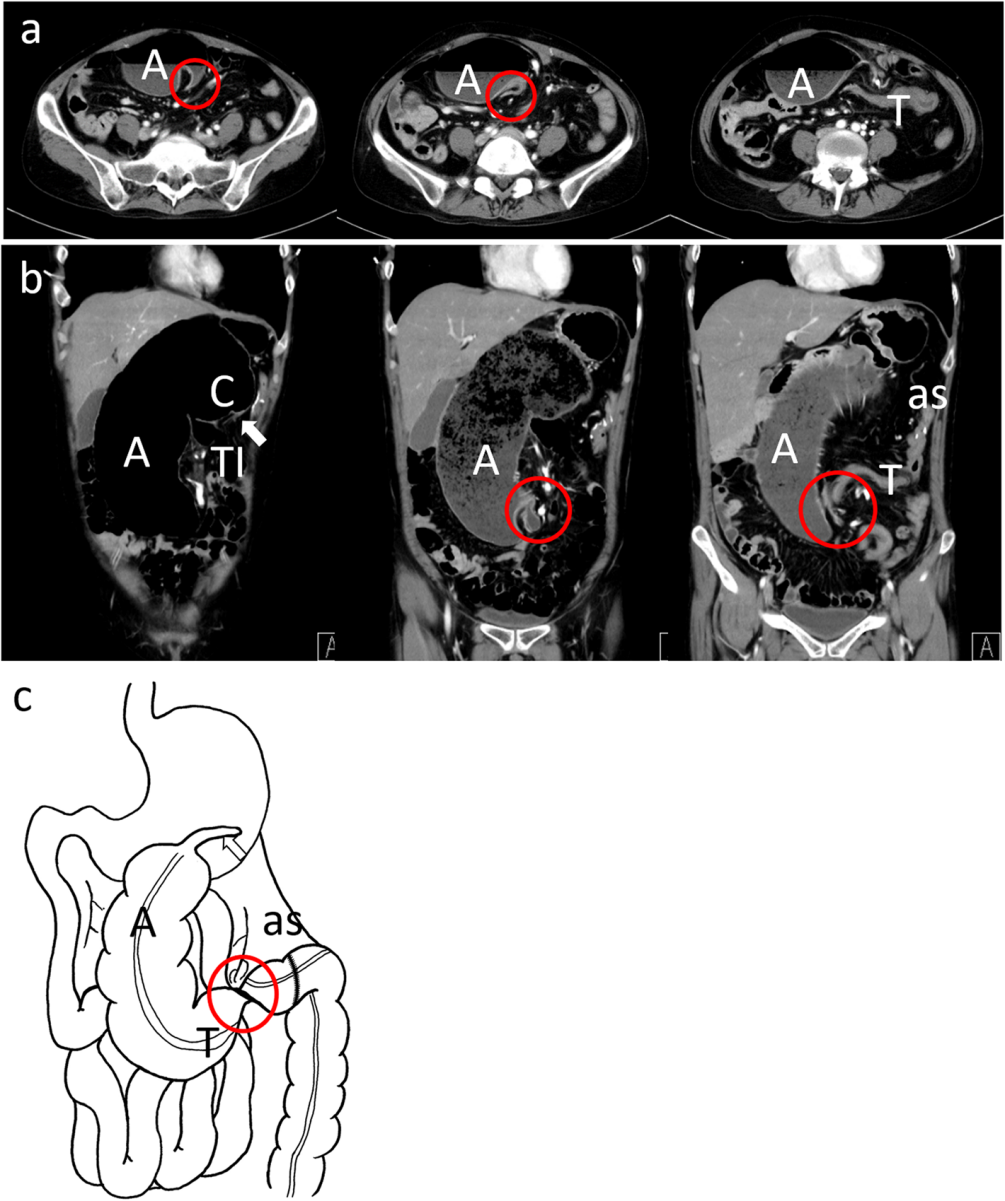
Enhanced computed tomography before the second surgery. Transverse section (a) and coronary section (b) images show the enlarged cecum (C) and ascending colon (A). The obstructive point was on the remaining transverse colon with a whirlpool sign (red circle). The anastomotic site of the first surgery (as) was placed in the left upper abdominal cavity. The appendix (white arrow) was in the upper abdominal cavity. The volvulus and the distribution of the gastrointestinal tract are indicated in (c)
